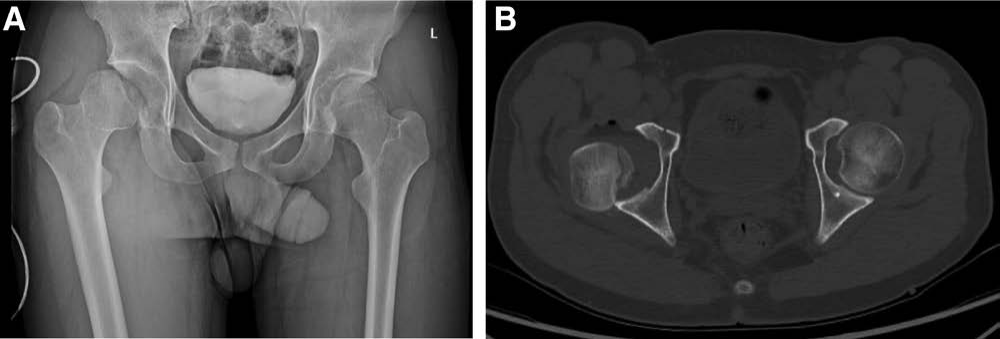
Initial image workup. (A): Anteroposterior hip x-ray showing right hip dislocation and femoral head fracture (pipkin type II). (B): Axial CT showing posterior dislocation of the right hip and a severely crushed femoral head. CT = computed tomography.
